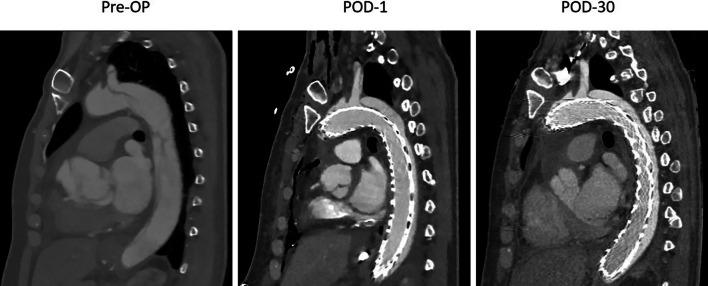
Computed tomography angioscanner showing the deployed AMDS in the true lumen at pre-op, POD1 and POD30
